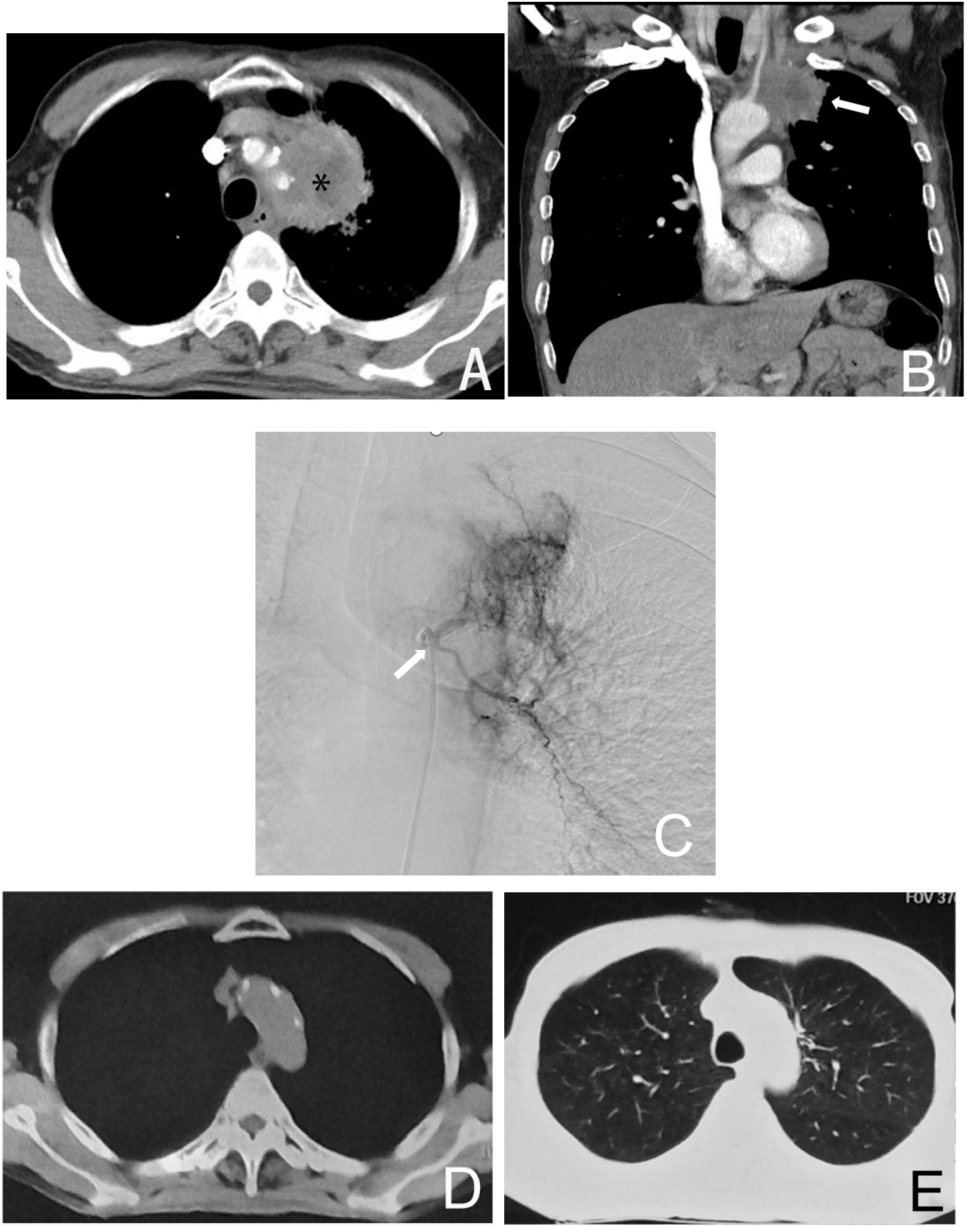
Male, 61 years old, diagnosed with left lung adenocarcinoma through a puncture biopsy, without driver mutations (no molecularly targeted therapy). Figure (A) shows the CT axial image of the chest, where the left upper lung cancer surrounds a major blood vessel, making it inoperable (*). Figure (B) shows the coronal image of the patient, indicating the left lung tumor (→). Figure (C) is a left bronchial arteriogram showing that the left lung tumor was supplied by the left bronchial artery with a rich blood supply (→). Figures (D, E) demonstrate that after four courses of BACE treatment, the tumor has disappeared, with the treatment achieving CR.
